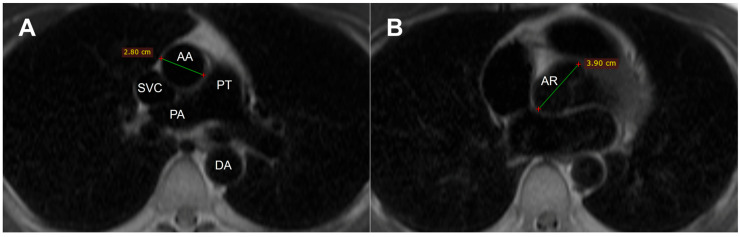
Aortic diameters were measured on axial magnetic resonance images at standardized anatomical landmarks using white-blood and black-blood MRI sequences. (A) Ascending aorta (AA) diameter measured at the level of the main pulmonary artery. (B) Aortic root (AR) diameter measured at the level of the sinuses of Valsalva. The green line indicates the measured diameter. AA: Ascending aorta; AR: Aortic root; SVC: Superior vena cava; PT: Pulmonary trunk; PA: Pulmonary artery; DA: Descending aorta.
